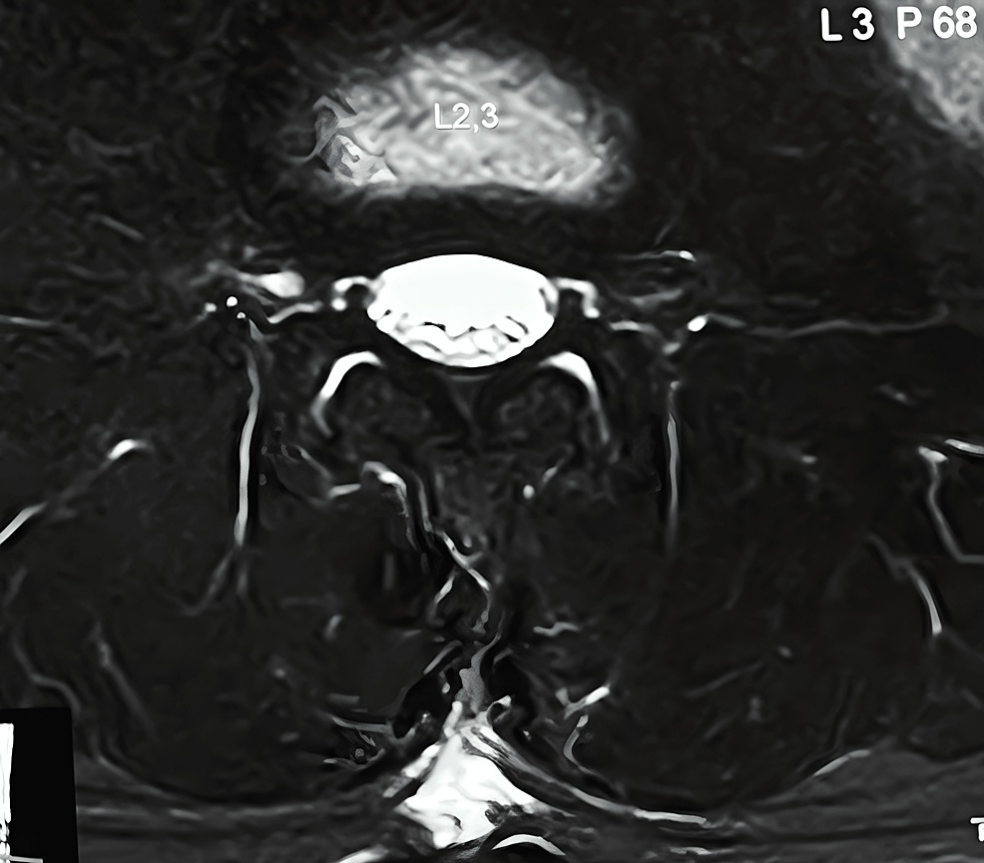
Follow-up MRI axial view Postoperative 18-month follow-up MRI axial view showing no structural changes in the spinal cord or nerve roots
